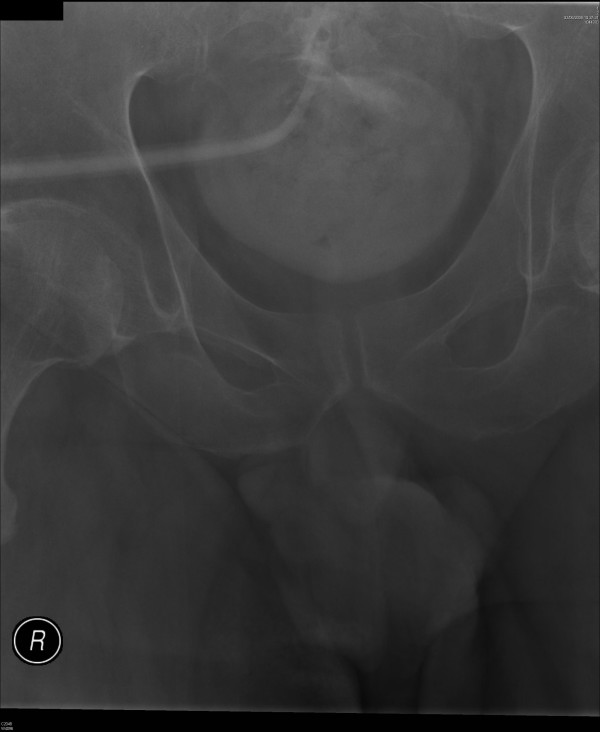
Following injection of 20 ml of Optiray 300 through suprapubic catheter, X-ray was taken. Cystogram showed that the tip of size 22 French Foley catheter had protruded beyond the dome of urinary bladder. There was suspicion of extravasation of small amount of contrast around the tip of Foley catheter.
